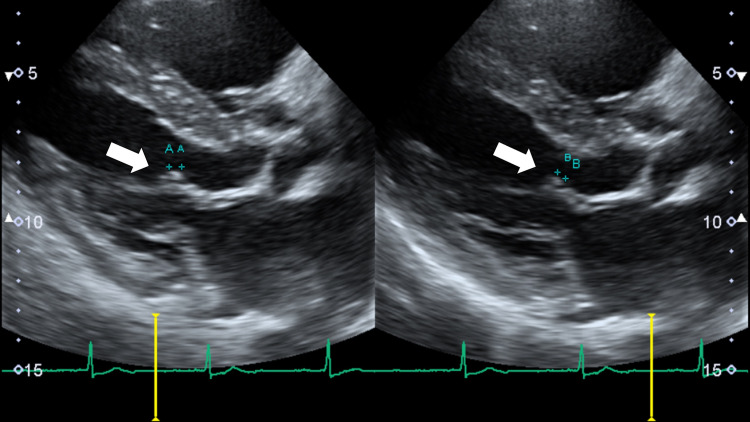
Transthoracic echocardiography on the fourth day of hospitalization revealing a 4–5 mm vegetation on the anterior mitral leaflet (white arrows pointing to the vegetation shown by + +)
